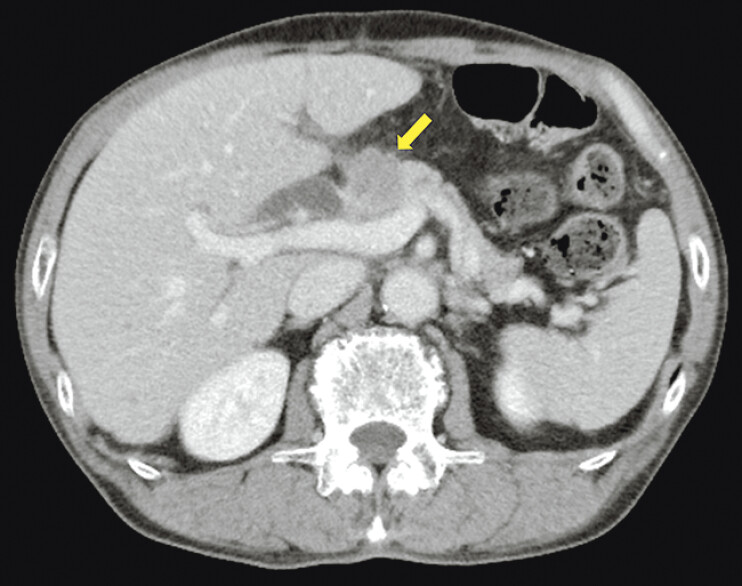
Contrast-enhanced computed tomography showing hilar lymph node metastases (arrow) from the gastric cancer.
